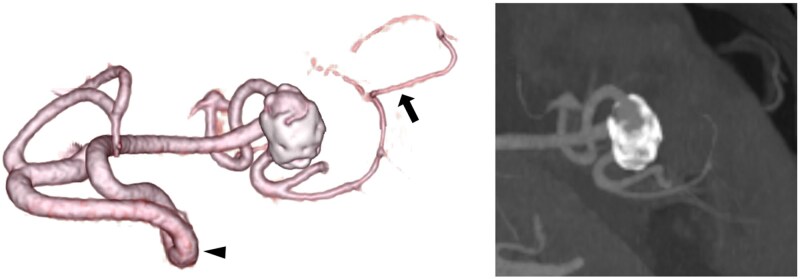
Contrast-enhanced computed tomography (CECT) before endovascular treatment. The volume rendering and maximum intensity projection reconstruction images show a wide-necked 18 × 10 × 10 mm splenic aneurysm. The mid-portion of the splenic artery exhibits significant tortuosity and angulation (black arrow head). The aneurysm is located at the bifurcation of the superior and inferior branches of the splenic artery. The inferior branch is connected to the left gastroepiploic artery (black arrow).
